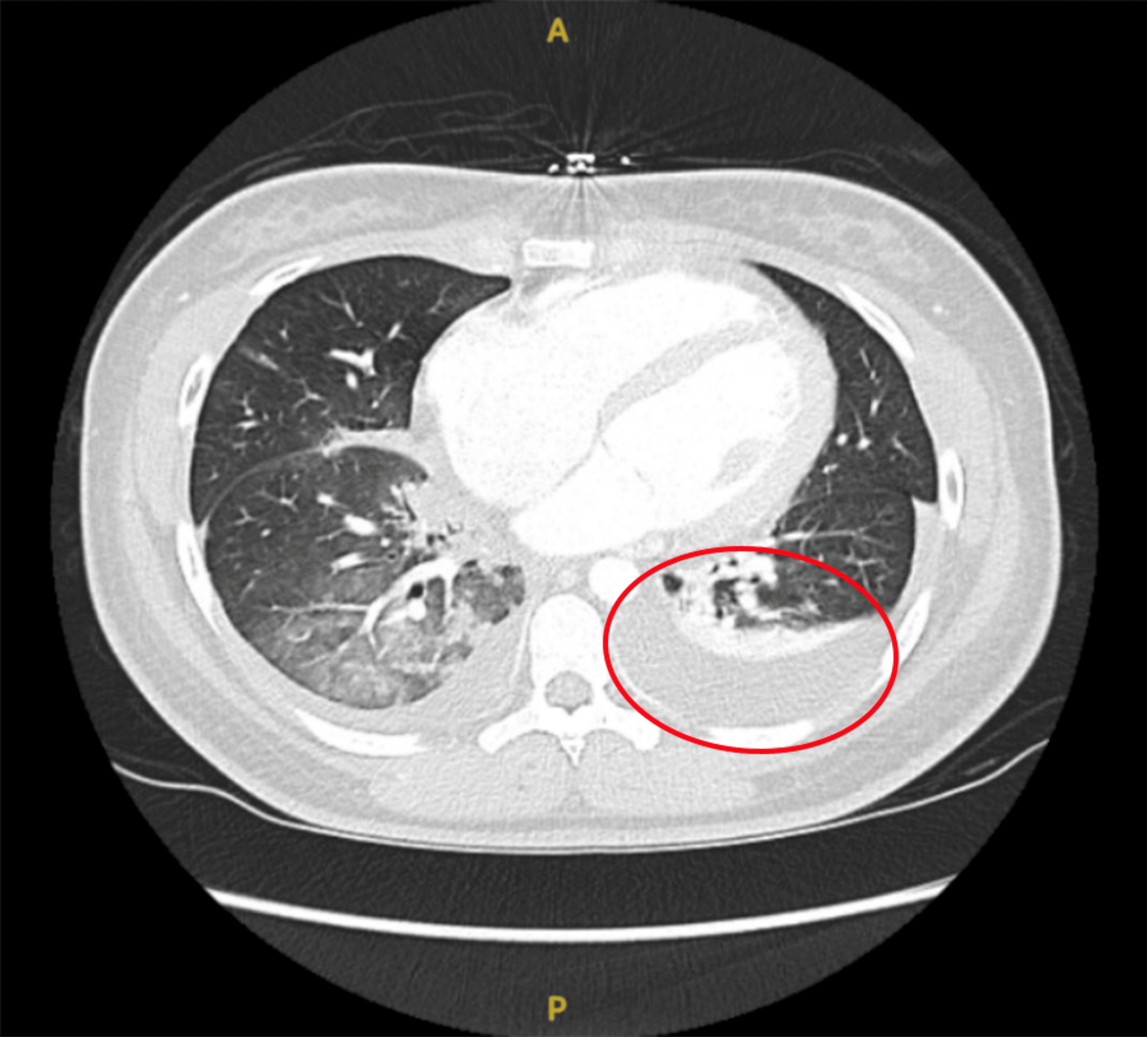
CT scan (transverse section) CT scan of the chest taken after diuresis and right thoracentesis shows bilateral pleural effusions, with fluid in both pleural cavities, more prominent on the right. The posterior right lower lobe (RLL) has consolidation (red circle), indicating lung tissue filled with liquid. There is also bilateral compressive atelectasis in the posterior segments, where lung tissue is collapsed due to the pressure from the effusions.
